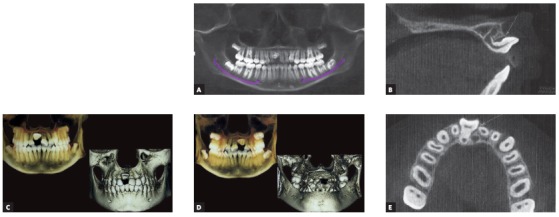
A) 3-D reformation: Tru-Pan view. B) Pretreatment CBCT image with 3-D reconstruction in sagittal plane, showing severely dilacerated root of the impacted incisor with a crown-root angle of 100^o^. C) 3-D reconstruction - labial view. D) 3-D reformation - lingual view. E) 3-D reconstruction - axial plane.
